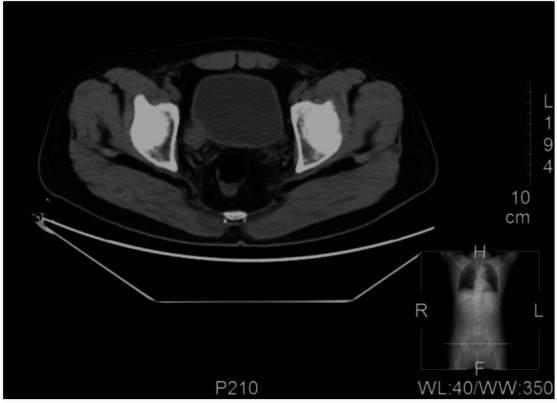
CT scan reveals a right lateral wall diverticulum of the bladder with a space-occupying lesion, measuring approximately 18 mm in diameter.
